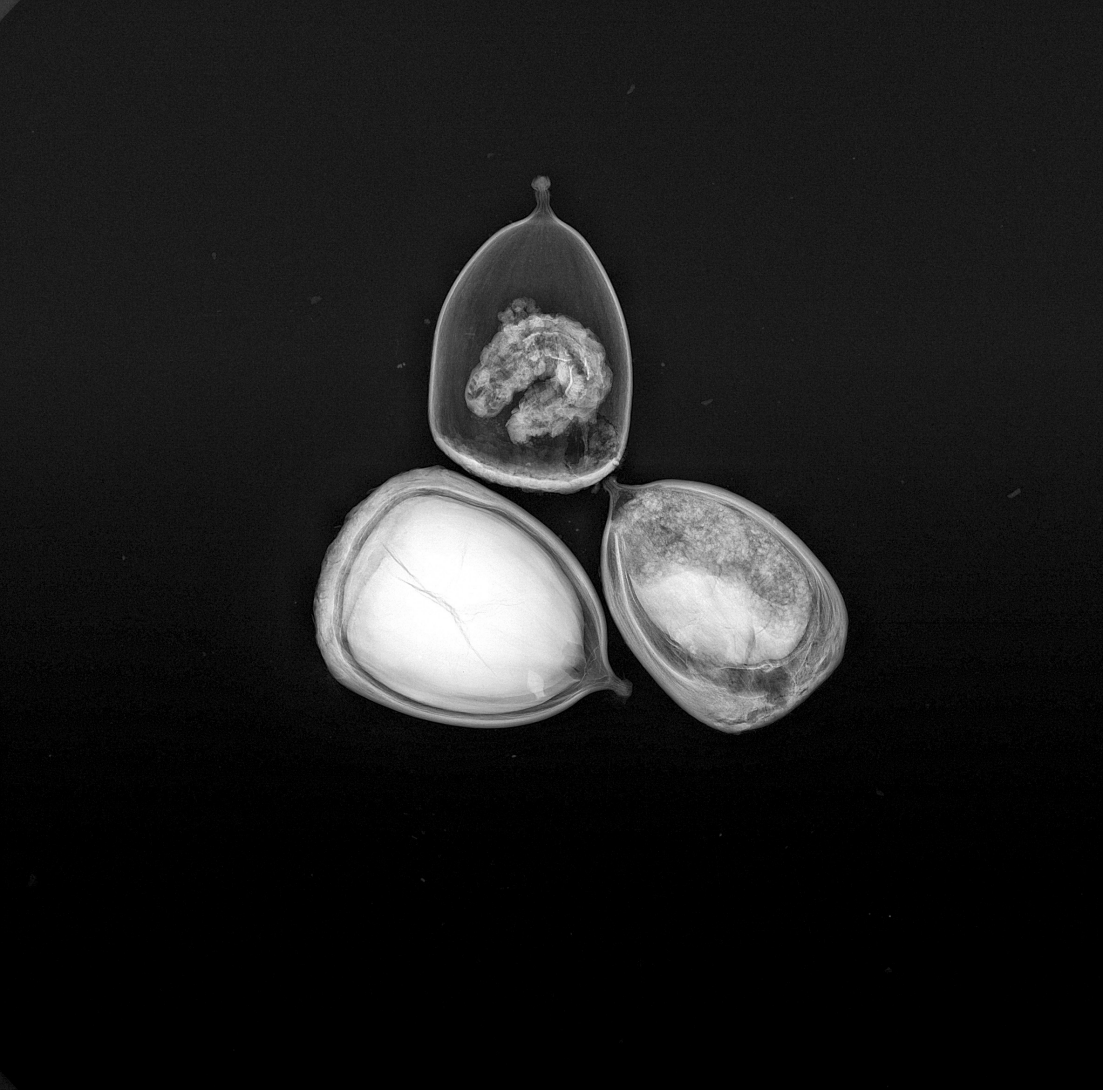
The radiograph of *Castanopsis megaphylla* collected from tropical XSBN in 2020. One intact (bottom left) and two damaged seeds, where a larva (top) and frass (bottom right) were detected, are shown here. The nuts are ~1 cm in length.
